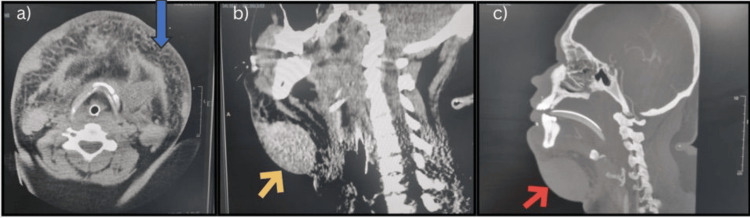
(a) Axial contrast CT showing subcutaneous high absorbance in the neck and bilateral submandibular area (blue arrow). (b) Sagittal contrast CT showing subcutaneous swelling and supraglottic narrowing (yellow arrow). (c) Sagittal contrast-enhanced CT showing extravasation (red arrow) in the lateral area of the right submandibular gland.
